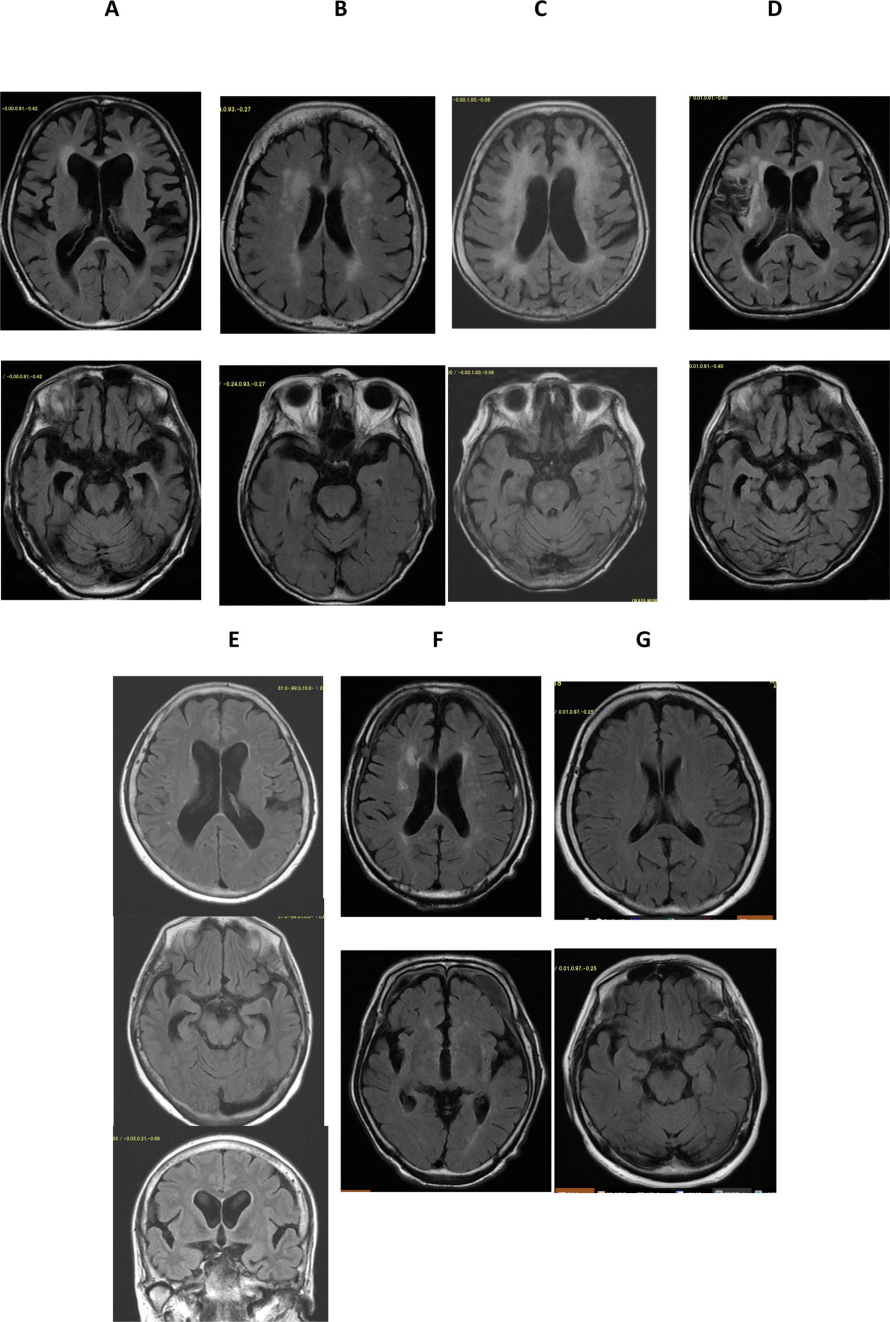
Representative brain MRI lesions in patients with HipFx. T2-FLAIR axial image. A. Alzheimer’s disease-type: significant atrophy of the para-hippocampal gyrus. B. Asymptomatic multiple ischemic cerebral lesions: multiple high signal intensity lesions in the white matter. C. Binswanger’s disease-type: extensive white matter lesions. D. Past symptomatic cerebral infarction: large low signal intensity lesion in the right temporal lobe. E. DESH (disproportionately enlarged subaracahnoid space hydrocephalus): enlargement of the ventricular and the Sylvian fissure F. Chronic subdural hematoma: crescent-shaped lesions between the dura and arachnoid G. Normal aging: no atrophy or no white matter lesions.
